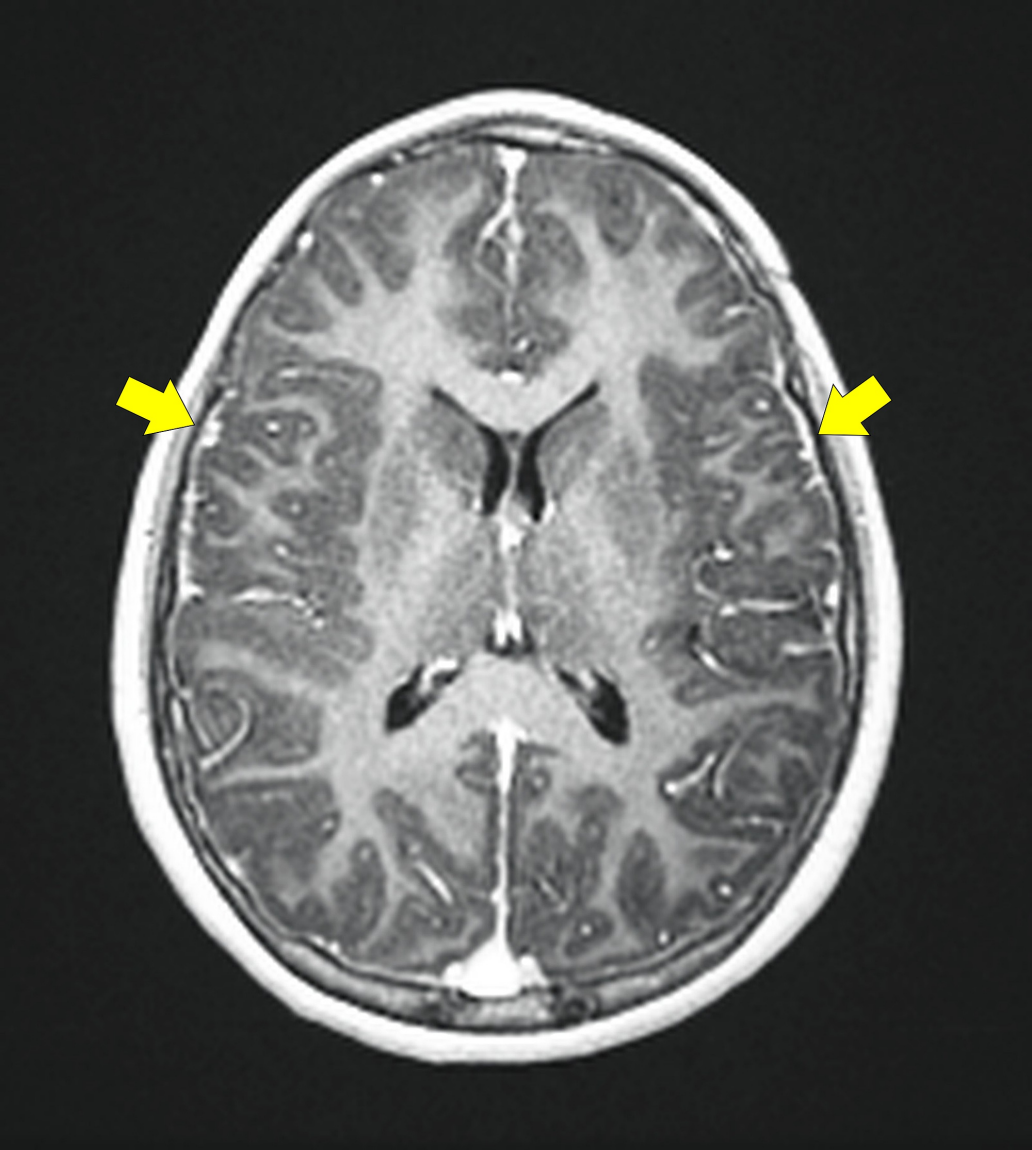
Axial post-contrast T1-weighted brain MRI showing diffuse leptomeningeal enhancement (yellow arrows) MRI: Magnetic resonance imaging.
